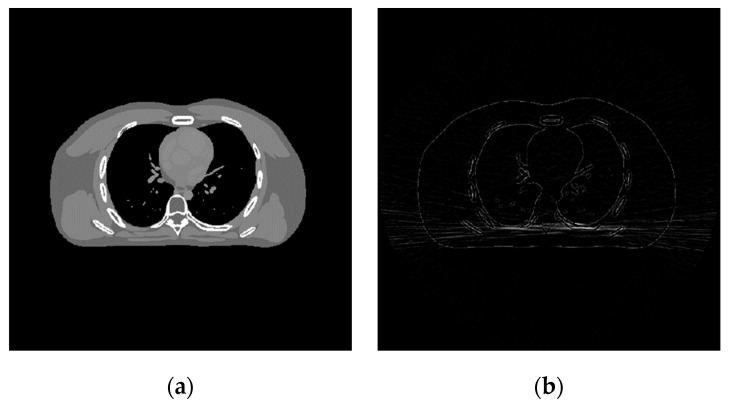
Training image. (a) Standard dose; (b) noise CT images.
